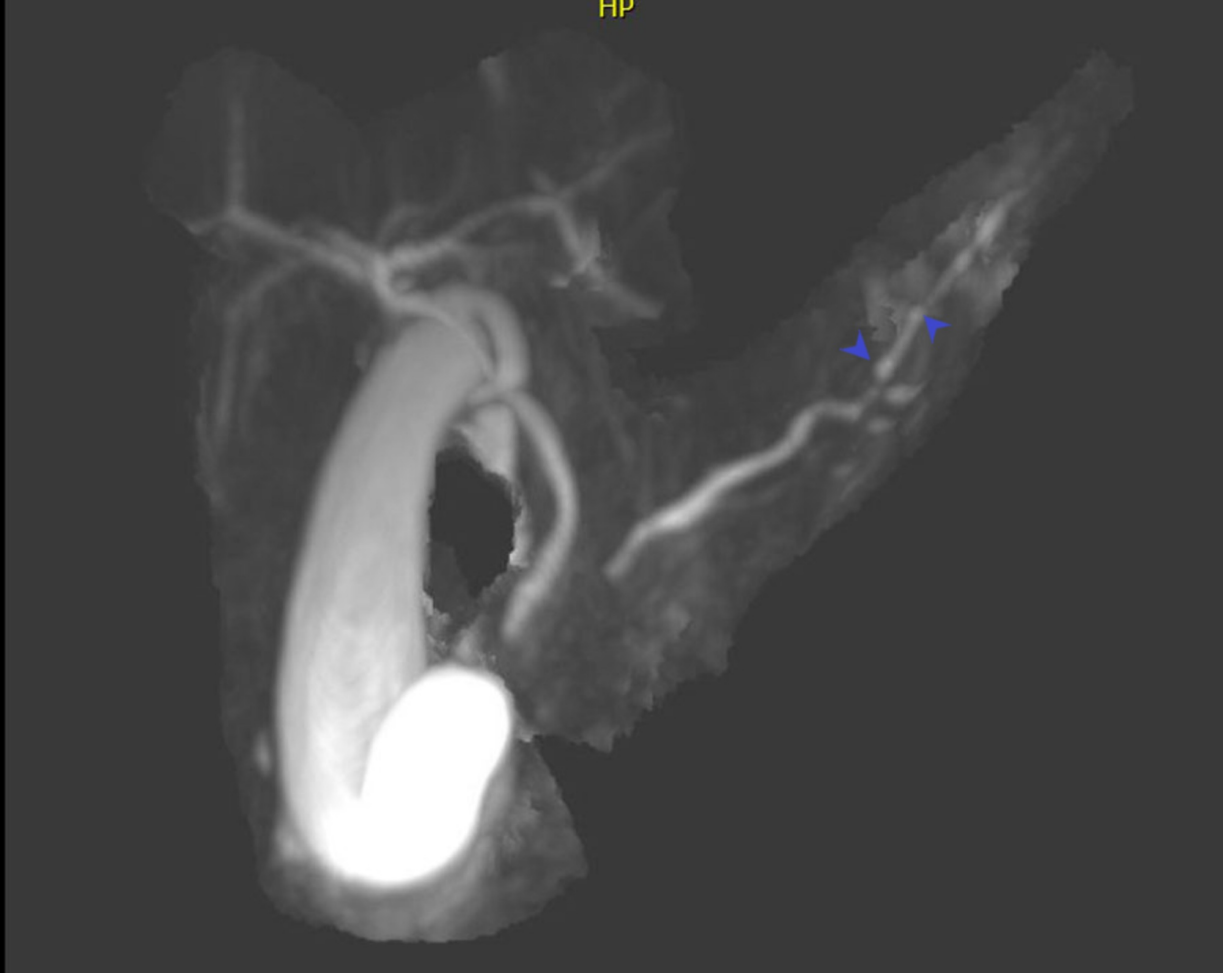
MRI of the pancreas after 3D processing. The image shows edematous pancreas and dilated pancreatic duct with beaded morphology (depicted by blue arrowheads).
